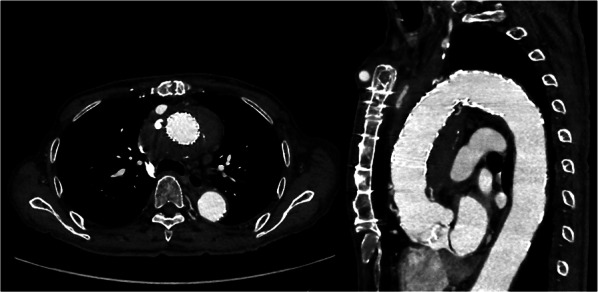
Postoperative computed tomography findings. Postoperative computed tomography shows no extravasation
